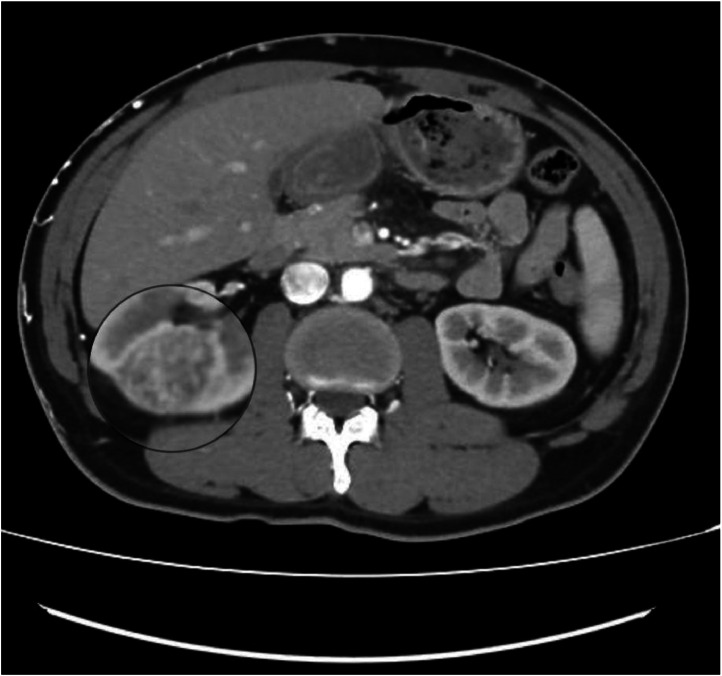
Coned view of a venous-phase CT at the level of the mid-portion of the right kidney, demonstrating a well-defined, heterogeneously enhancing renal cortical lesion. These imaging features are suggestive of either renal cell carcinoma or metastatic deposits. (However, no further investigations were conducted to confirm the diagnosis).
